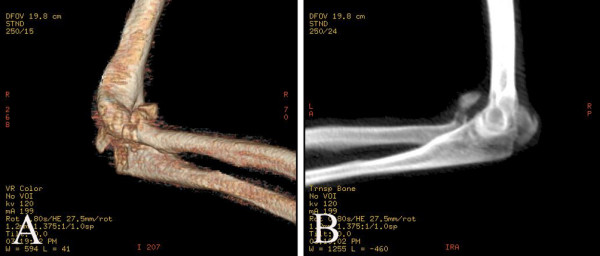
3D CT of posterior elbow dislocation with fracture of the olecranon and radial head, without coronoid fracture.
